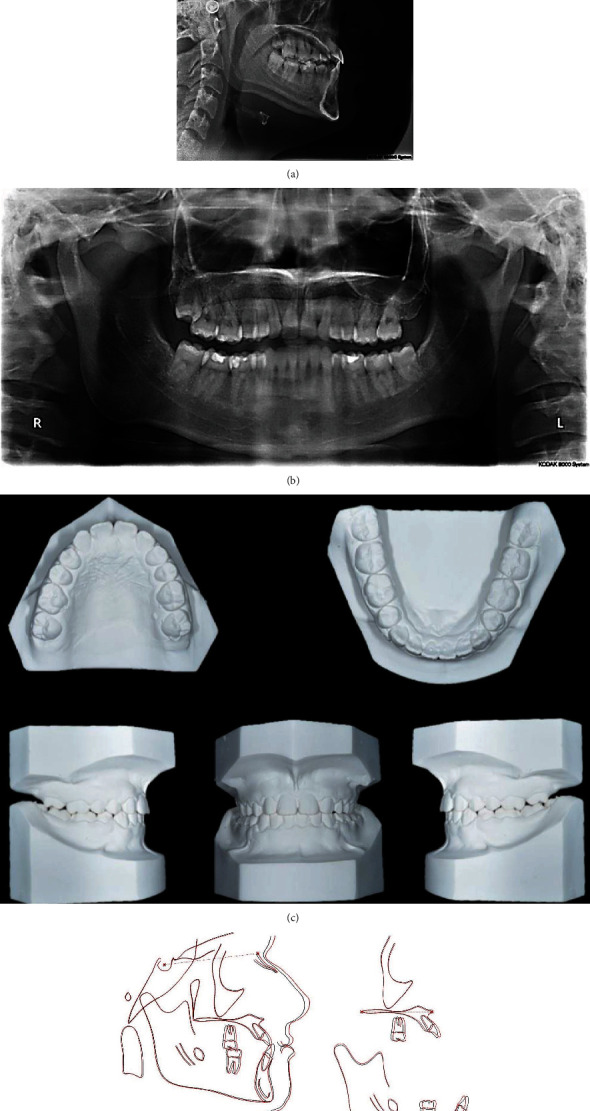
Posttreatment cephalometric radiograph, panoramic radiograph, study models, and superimposition: (a) cephalometric radiograph, (b) panoramic radiograph, (c) study models, and (d) cephalometric superimposition.
